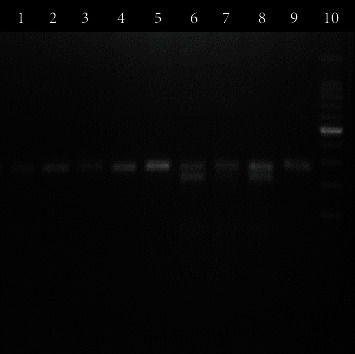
PCR-RFLP of IL-4 (590 C/T) SNP by BsmFI restriction enzyme. Lanes 1, 2, 3, 4, 5, and 9 show TT genotypes (252 bp), and lanes 6, 7, and 8 show CT genotypes (252 bp, 192 bp). Lane 10 shows a 100 bp DNA ladder.
